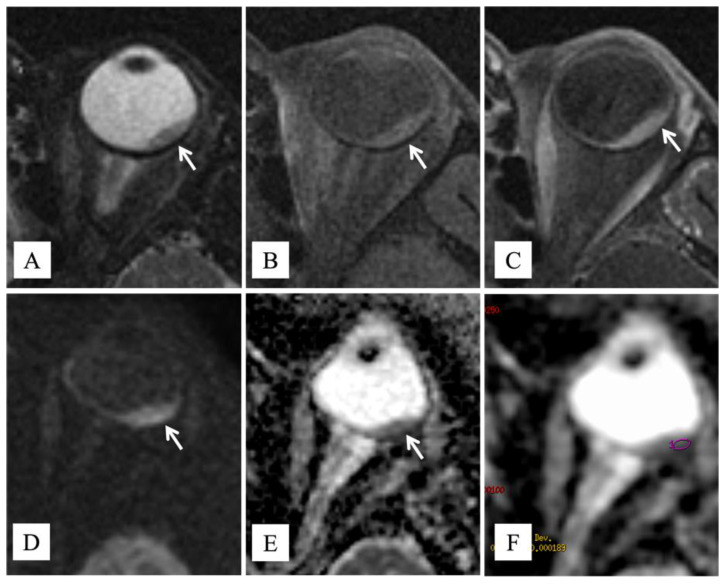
MR findings. Axial (A) T2-weighted turbo spin-echo STIR and (B) fat-suppressed T1-weighted images show a lentiform-shaped intraocular mass along the postero-lateral aspect of the left globe (white arrows), adjoining the optic disk. The lesion exhibits intermediate signal intensity on both T1- and T2-weighted images, a finding consistent with poorly pigmented melanoma. On (C) axial contrast-enhanced fat-suppressed T1-weighted image, the neoplasm demonstrates marked enhancement (white arrow). On (D) axial DW image (b = 1000 s/mm^2^) and (E) corresponding ADC map, the tumor displays restricted diffusion with high signal intensity on DWI image and low signal intensity on ADC map (white arrows), a finding consistent with hypercellularity. (F) The ADC value was 840 × 10^−6^ mm^2^/s.
